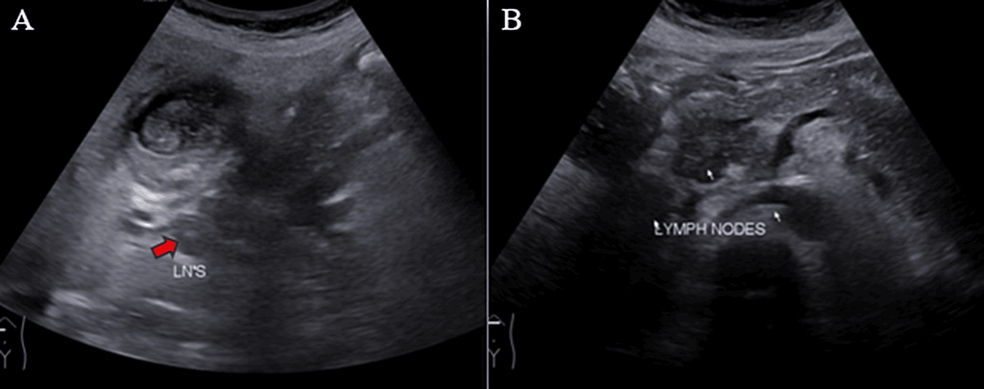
Ultrasonography images reveal enlarged necrotic lymph nodes in the periportal region (red arrows) (A) and retroperitoneal region (white arrows) (B). LN'S: Lymph nodes
